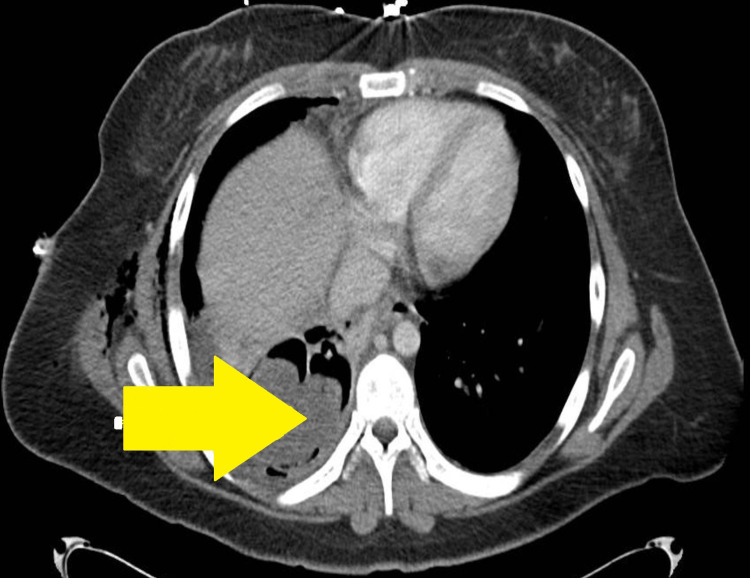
CT chest (yellow arrow indicates a lesion).
